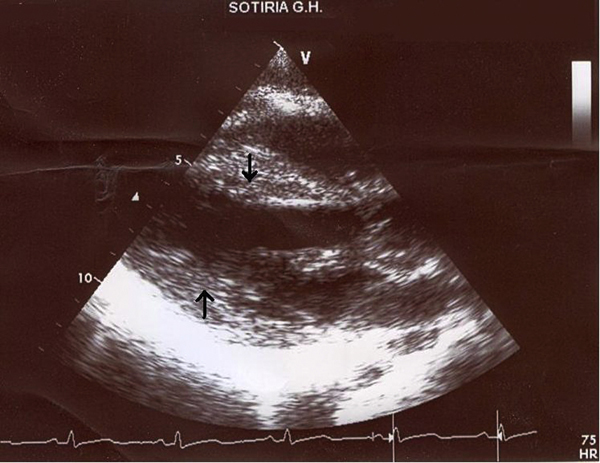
Echocardiogram, parasternal long axis. Arrows indicate the swelling of the intraventricular septum and the left ventricular posterior wall.
